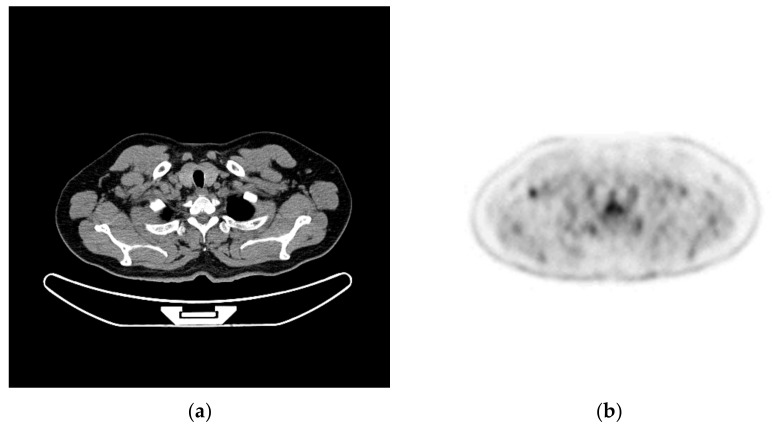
Registration of PET and CT. (a) CT image. (b) Enhanced PET image.
